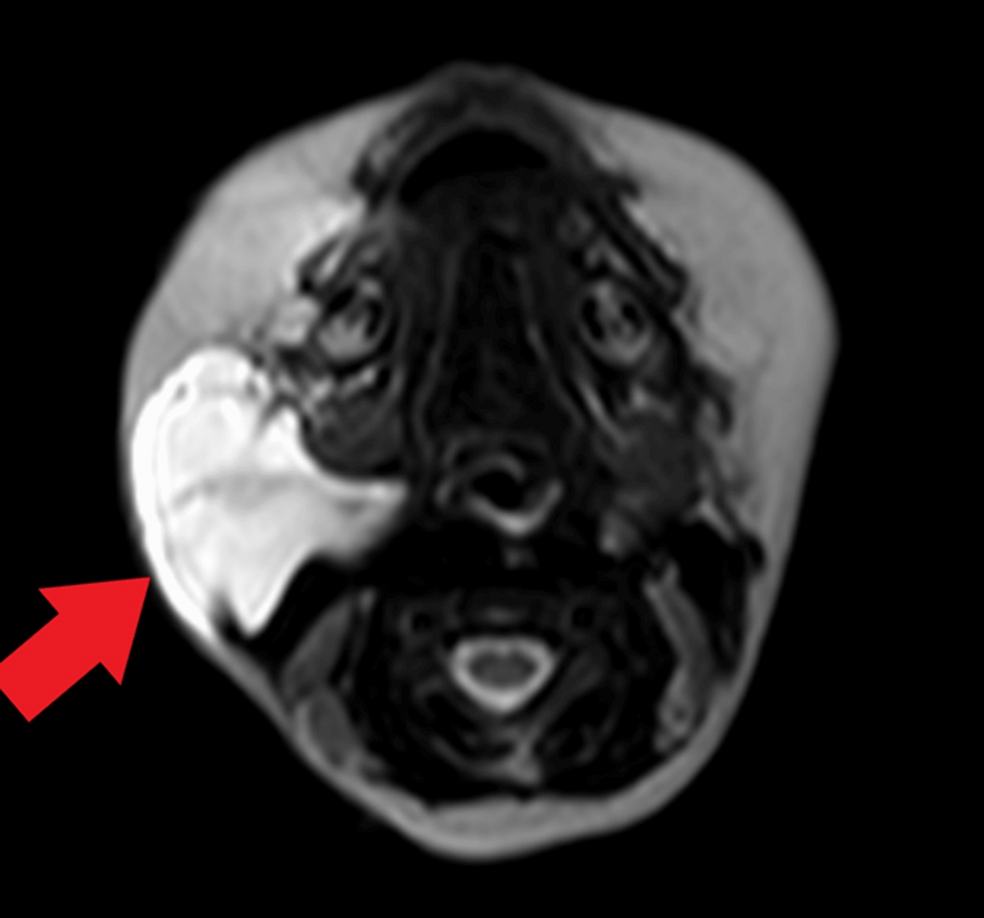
Magnetic resonance imaging revealing cystic lesion in the right submandibular region appearing hyperintense on T2-weighted imaging (red arrow).
